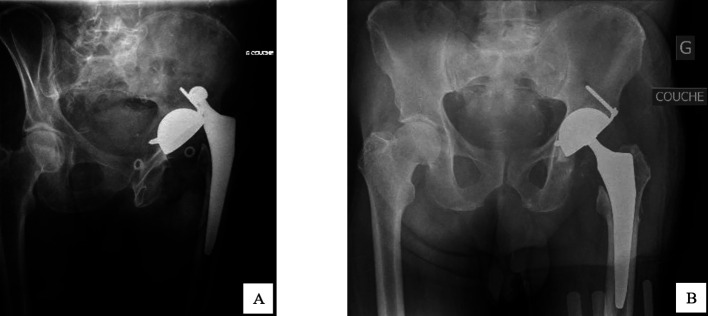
Radiographs (A) and (B) show dislocation and postoperative revision with DMC Novae-E TH, respectively.
